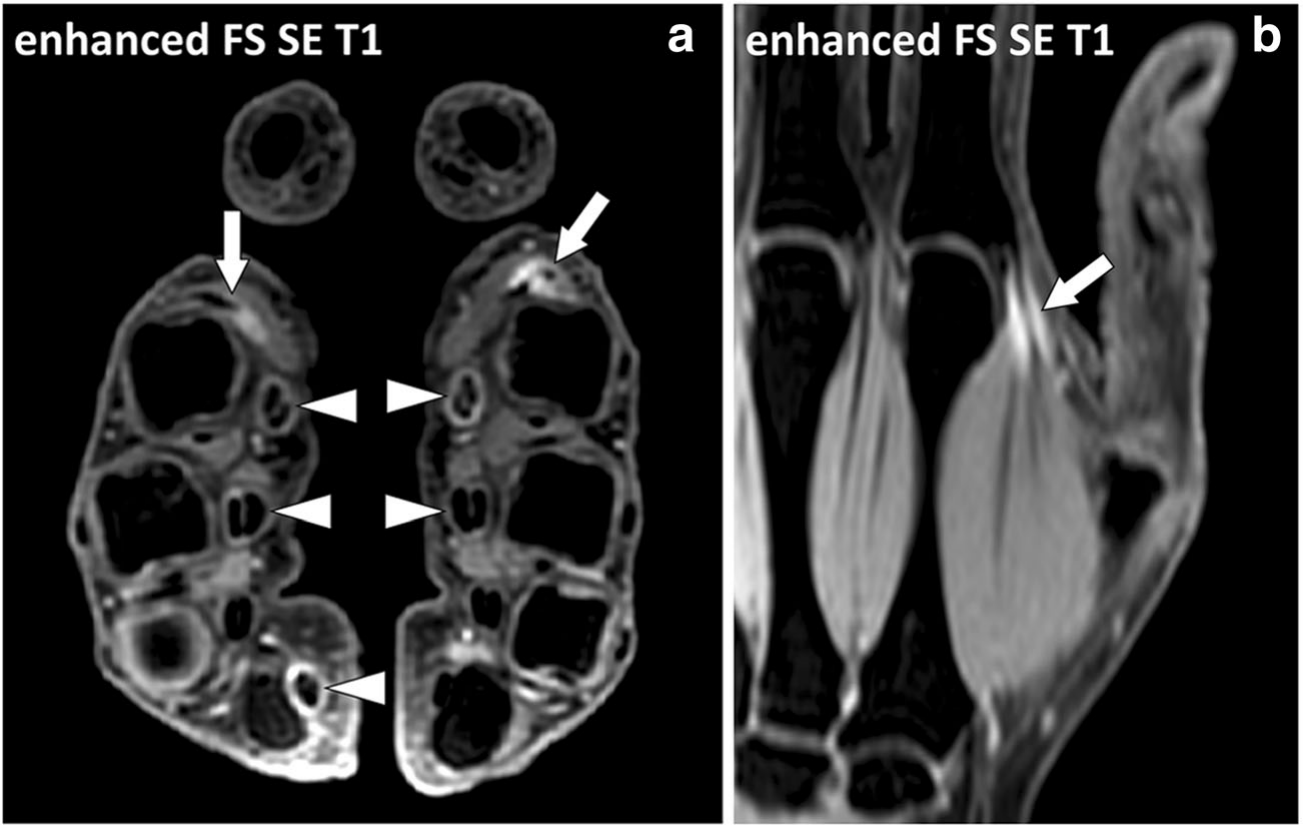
Contrast-enhanced fat-suppressed T1-weighted (a) axial image of the hands and (b) coronal reformat of the left hand of a 30-year-old female with seropositive non-erosive rheumatoid arthritis. MRI demonstrates inflammatory changes of the distal myo-tendinous junctions of the first dorsal interosseous muscles (arrows). Lack of synovitis suggests primary inflammatory involvement of the myo-tendinous junction. Tenosynovitis of the flexor digitorum tendons is also seen (arrowheads)
